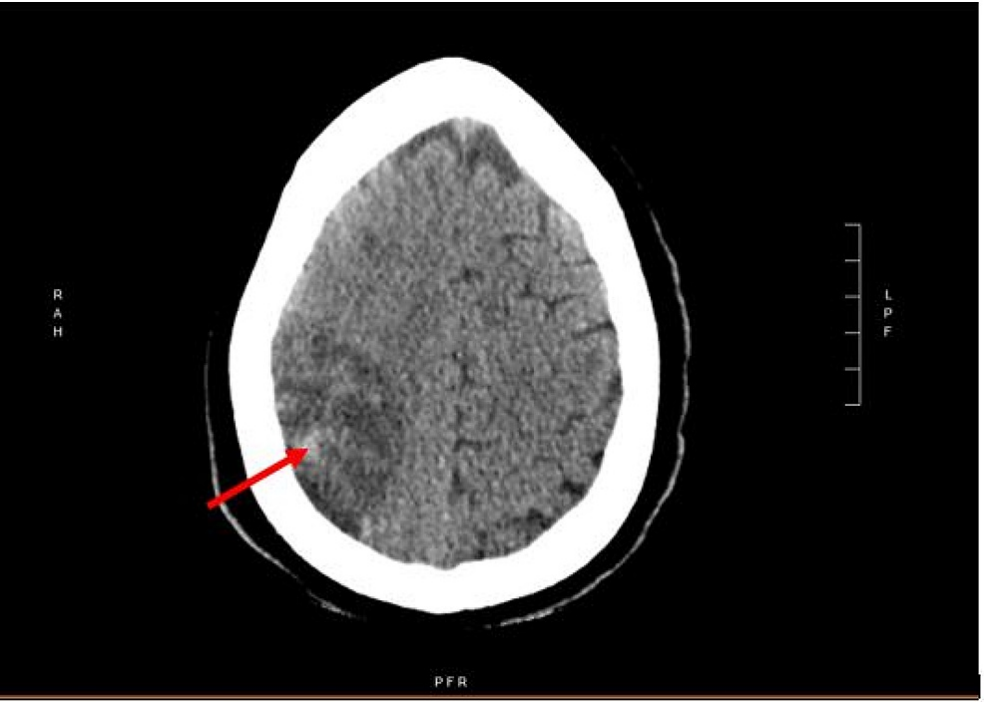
Repeat noncontrast head CT demonstrating an interval sulcal and right basal ganglia infarction and hyper-attenuation (arrow) consistent with petechial hemorrhage.
